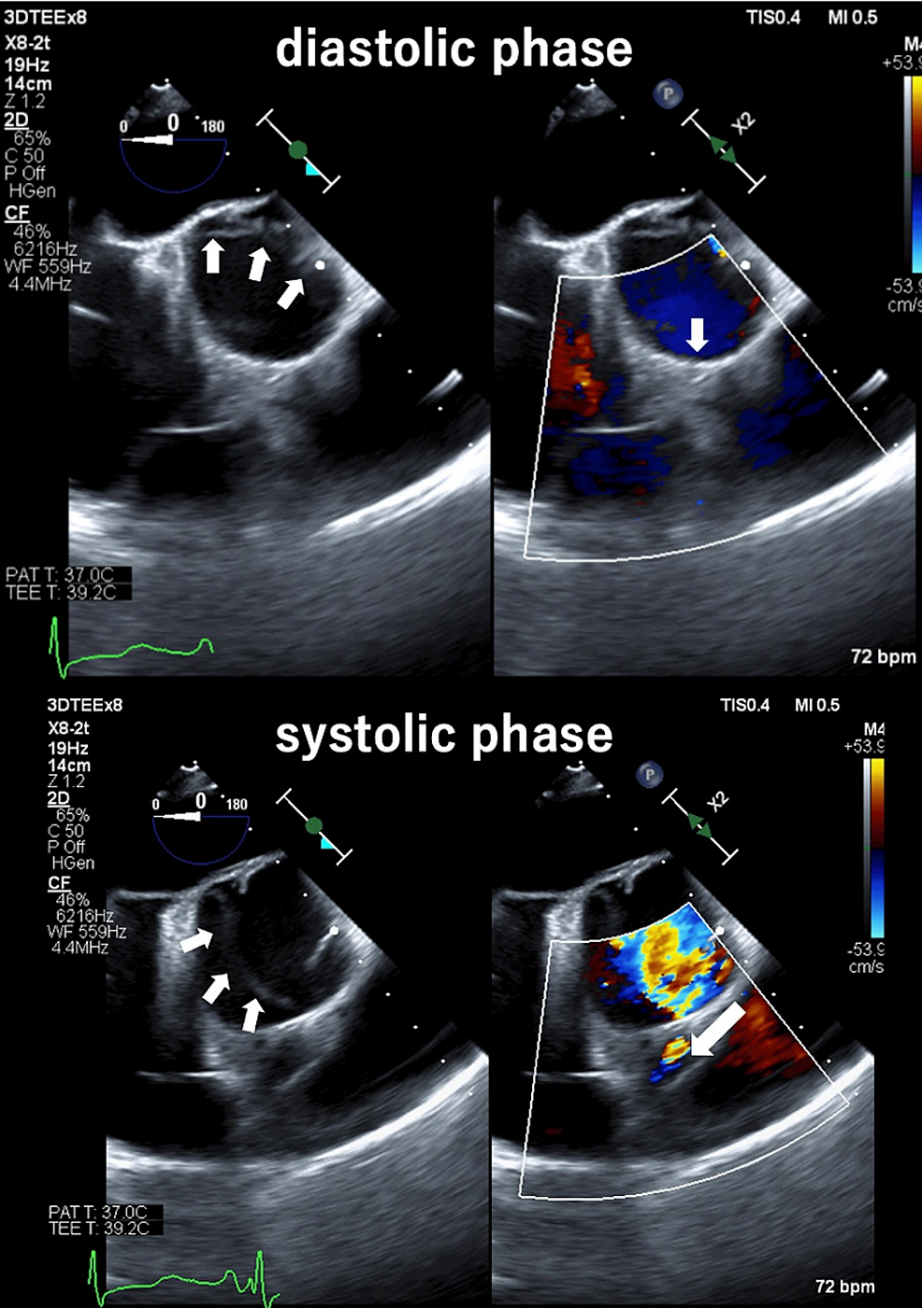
Transesophageal echocardiographic images after induction of anesthesia (aortic valve level, short axis view: left panel: B-mode, right panel: color Doppler). The right coronary artery origin was occluded during diastole by the aortic flap (upper row). Arrows in upper left panel: aortic flap; arrow in upper right panel, right coronary artery origin. The right coronary artery origin was occluded during the diastolic phase by the aortic flap, but this was released during the systolic phase (lower row). Arrows in lower left panel: aortic flap, arrow in lower right panel: direction of blood flow in the right coronary artery.
